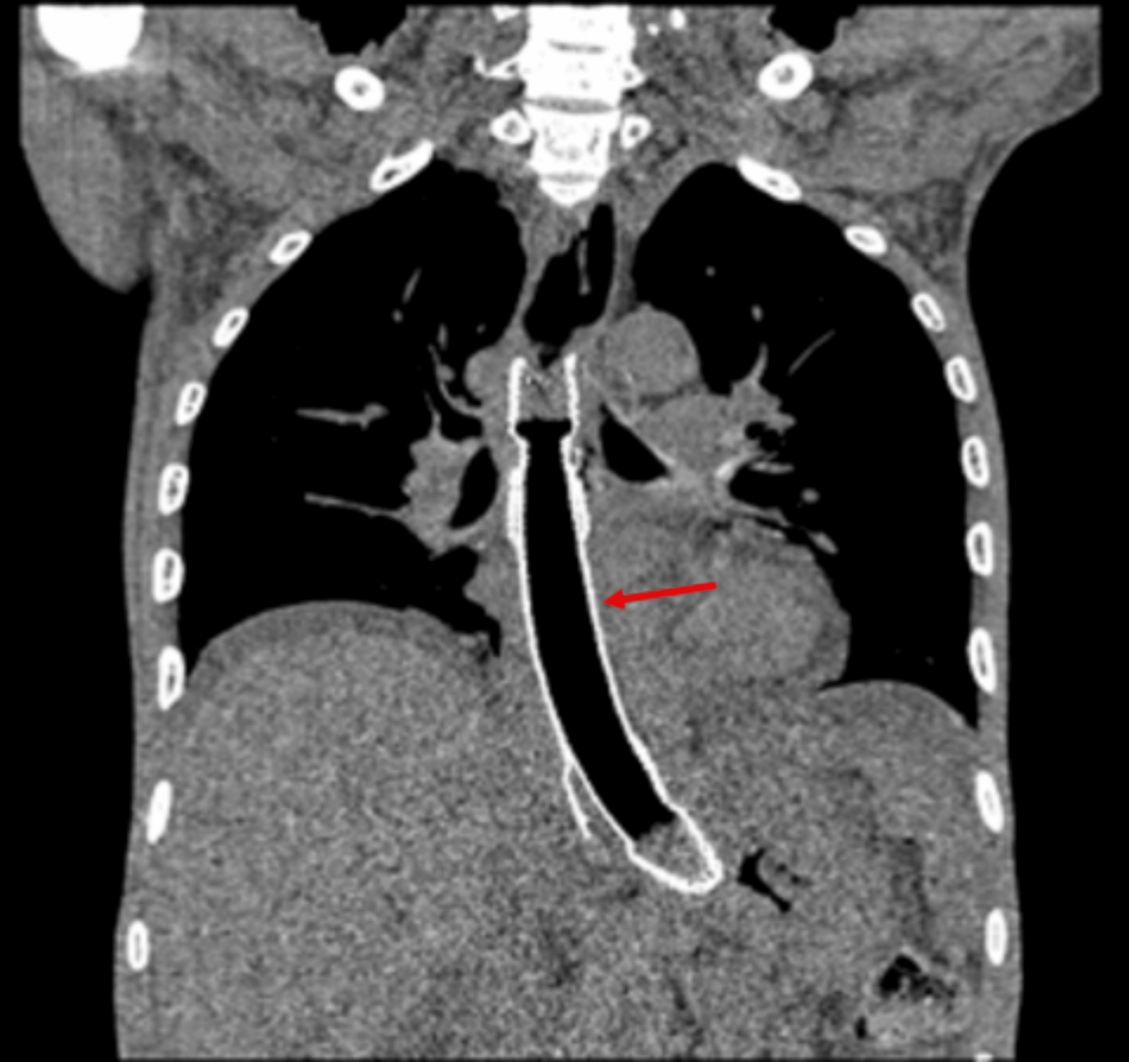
Plain CT - coronal section The red mark shows the esophageal stent in situ at the level of D4-D11 vertebral bodies.
